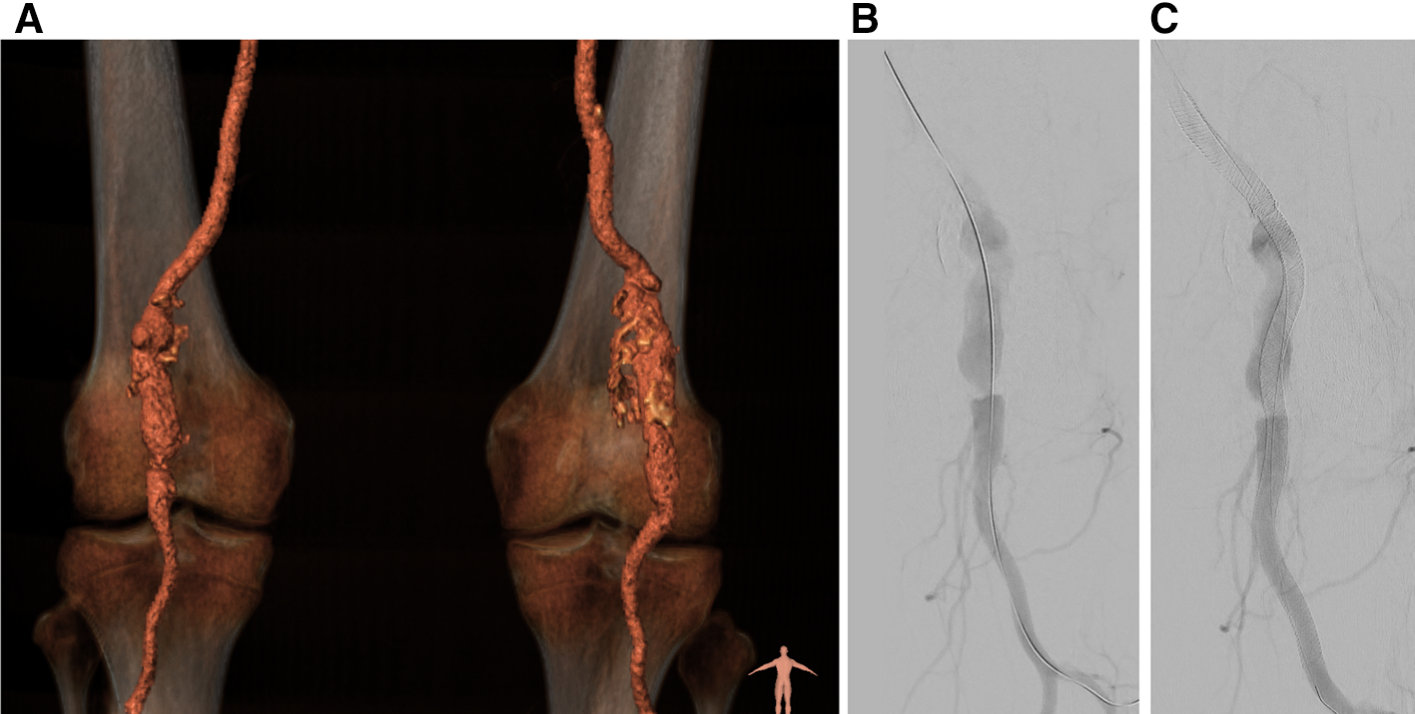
Clinical case example of a treated popliteal aneurysm. A posterior view of CTA showing bilateral popliteal aneurysm with curved inlet. B and C DSA of right popliteal aneurysm before (B) and after (C) treatment. Video 1 shows the telescoping deployment of the Supera stent
